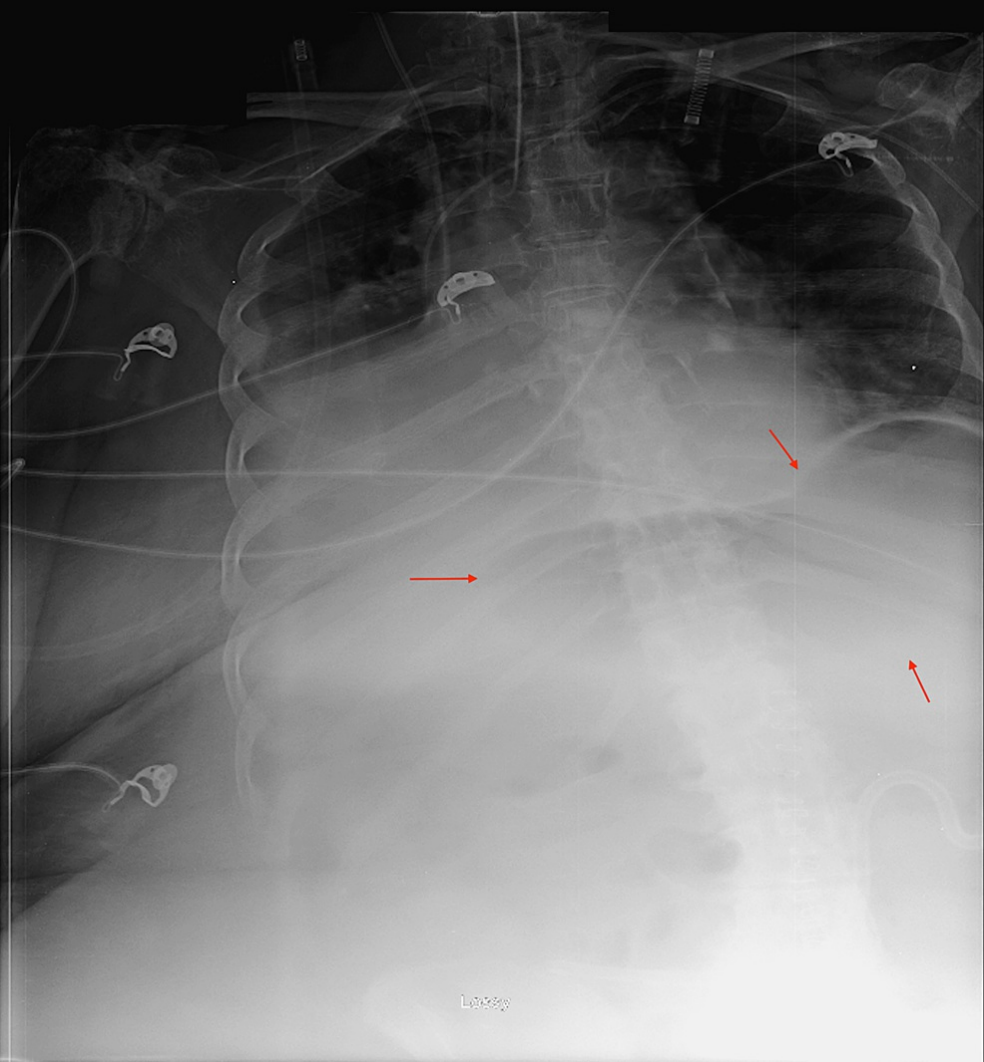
Upright abdominal radiograph demonstrating gaseous distention of the stomach with no free air identified
